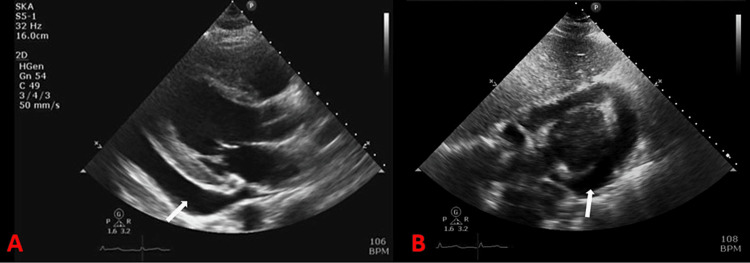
Transthoracic echocardiogram - parasternal long-axis (A) and subcostal (B) views demonstrating large pericardial effusion (white arrows).
